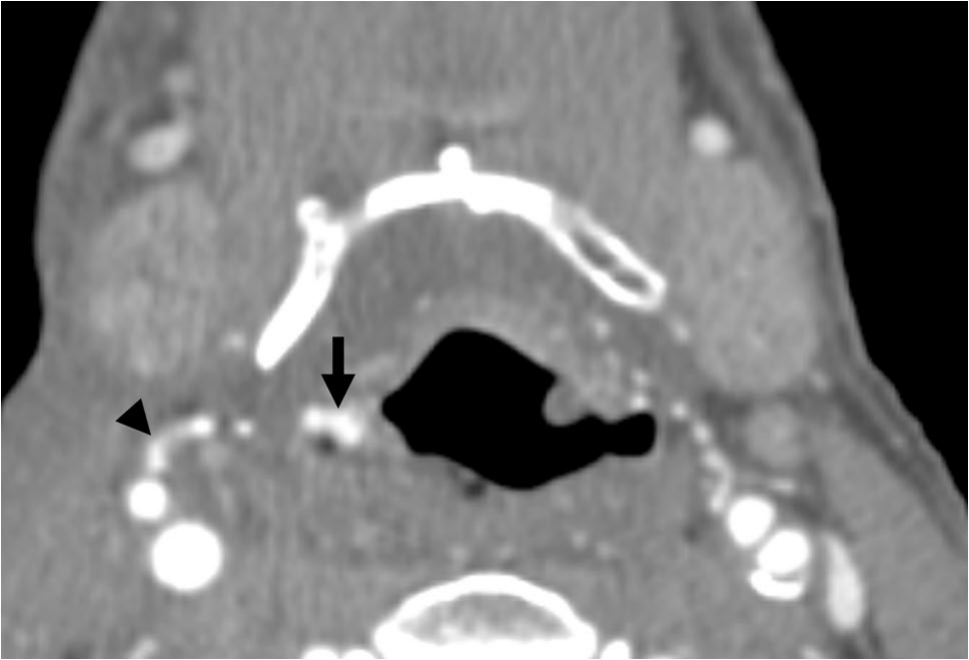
Patient 1. Axial postcontrast CTA image of the neck at the level of the pyriform sinuses demonstrates linear contrast material in the right pyriform sinus (black arrow), which appears to extend laterally towards an irregular appearing infrahyoid branch of the superior thyroidal artery (black arrowhead)
